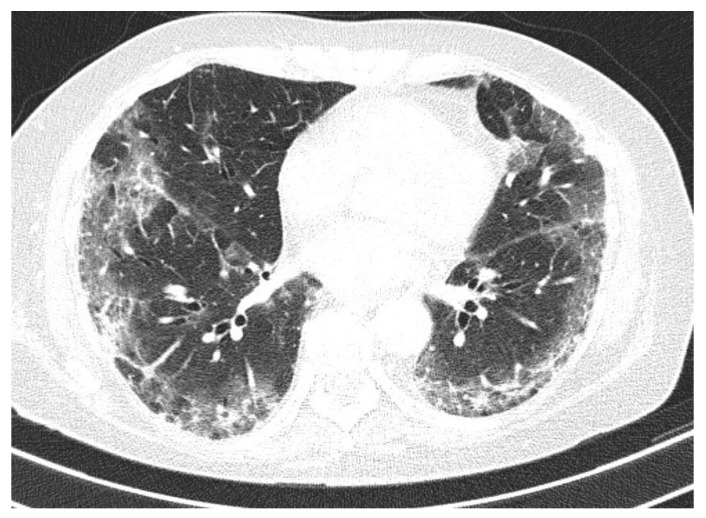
HRCT of a patient with AE-ILD in the course of RA-ILD.
